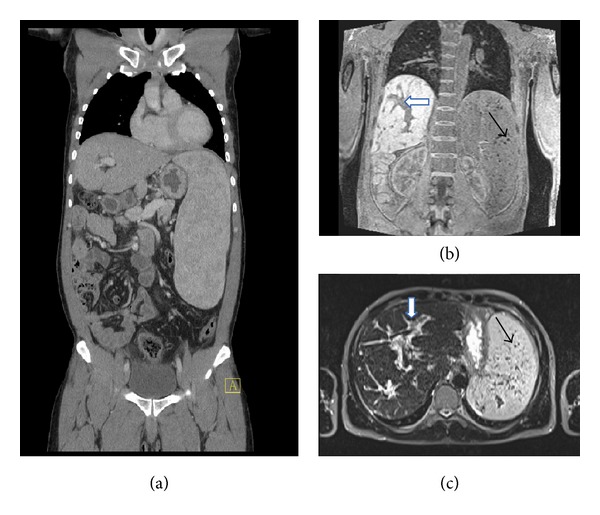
(a) Computerized tomography (CT) imaging showing the enlarged spleen (b) and (c) magnetic resonance imaging (MRI) demonstrating the periportal fibrosis (white arrows) and the Gamna-Gandy bodies (siderotic nodules) in the spleen (black arrows).
